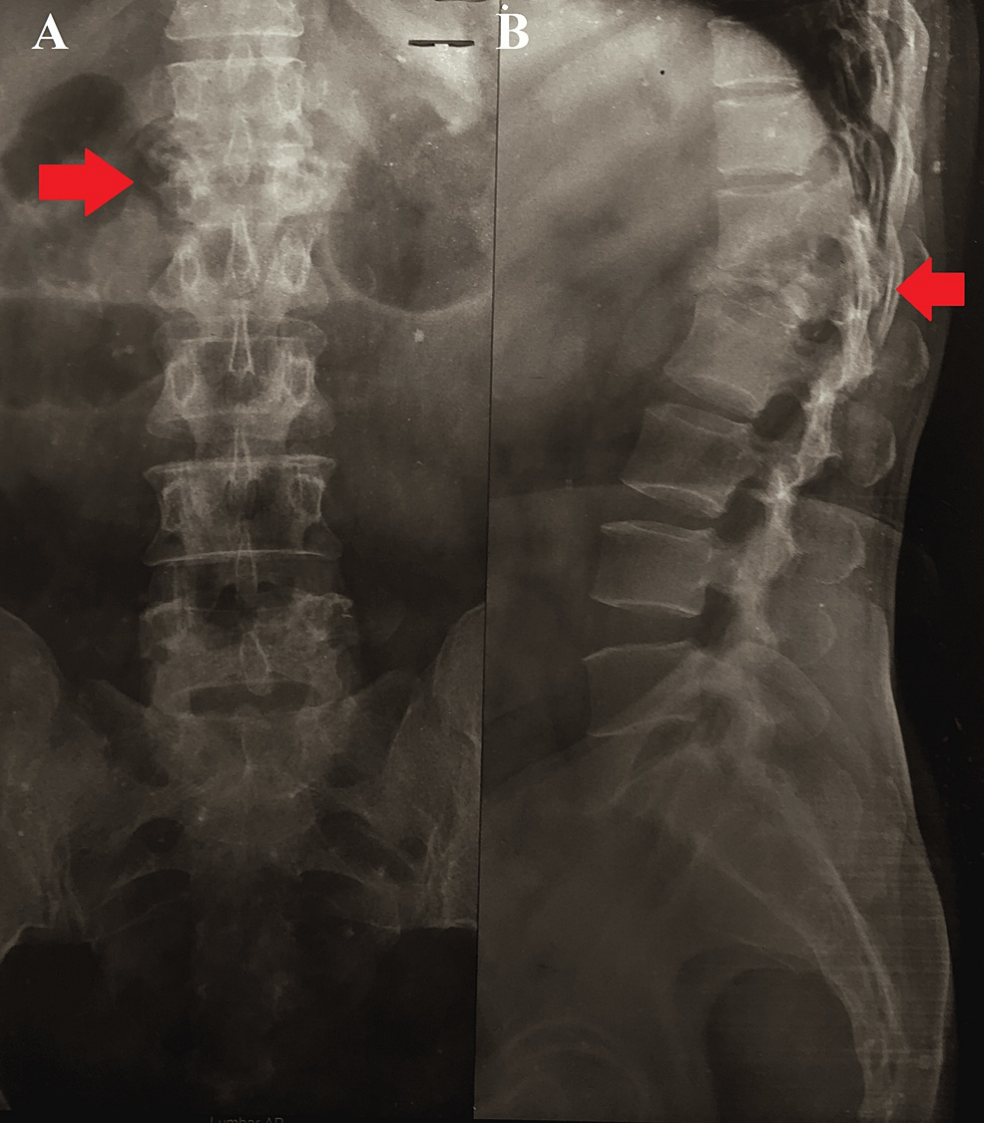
Plain radiograph of the lumbar region A: AP view, B: Lateral view
